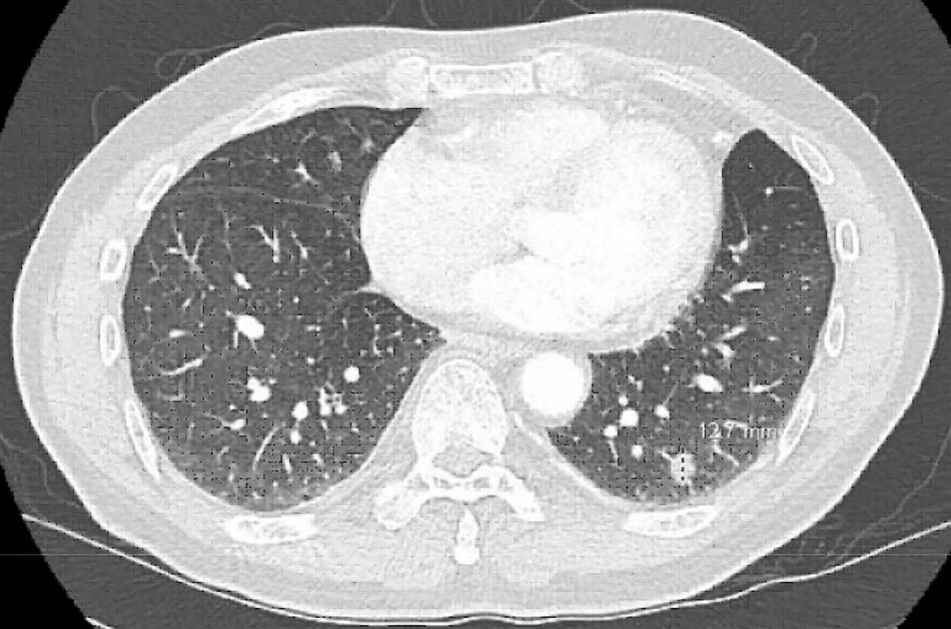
CT scan demonstrating a spiculated left lower lobe nodule.
